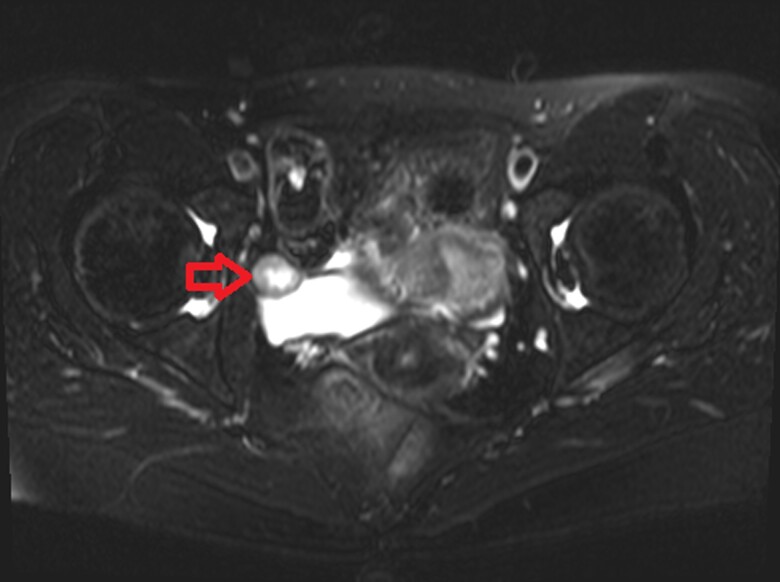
Magnetic resonance imaging pelvis with and without gadolinium. 1.9 × 1.6 × 1.3 cm well-circumscribed, heterogeneously T2 hyperintense, T1 isointense to muscle, enhancing lesion with peripheral diffusion restriction, and central cystic degeneration is seen within the right superior lateral aspect of the bladder wall.
